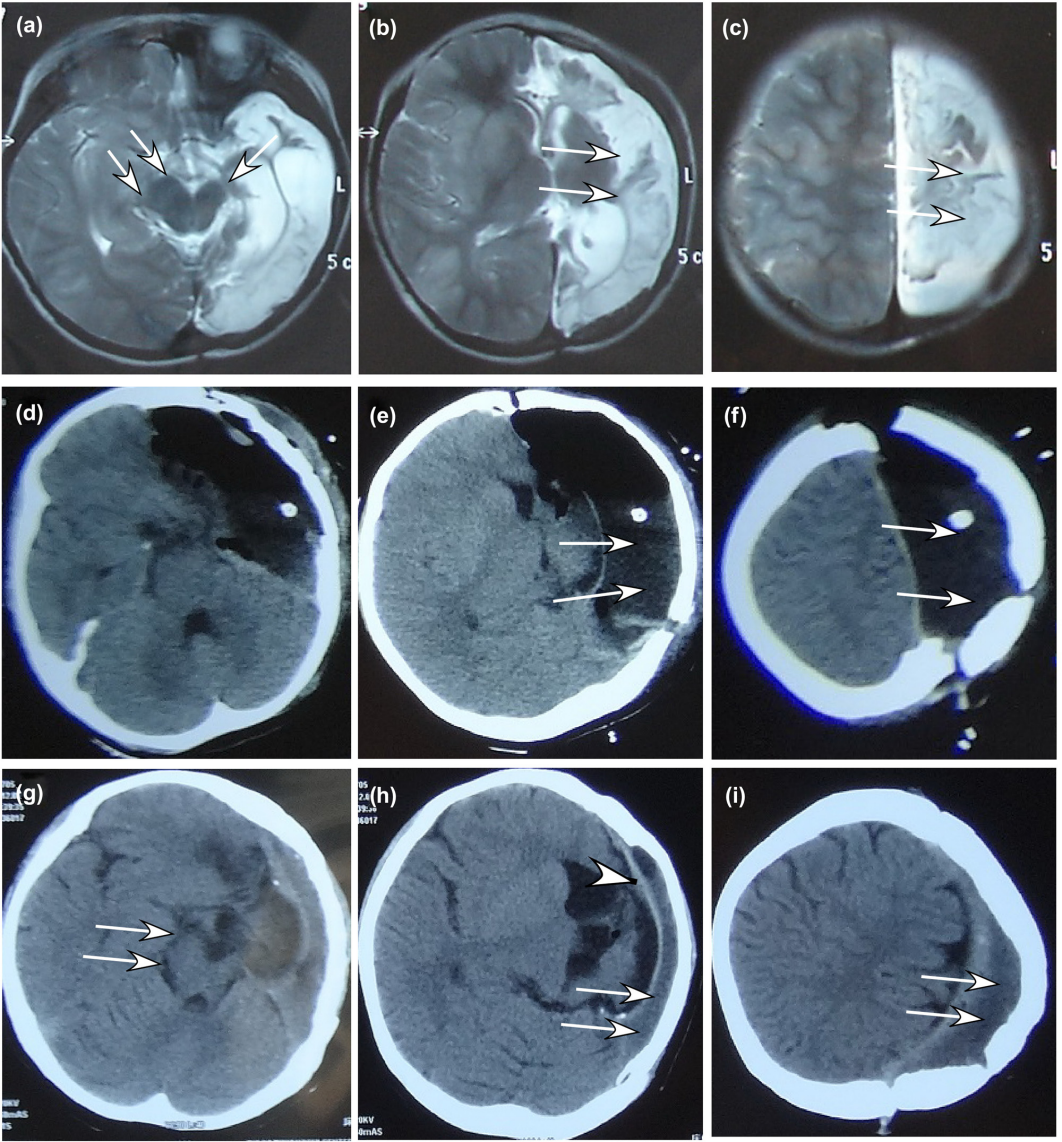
An 8-year-old girl with infantile hemiparesis and medically intractable epilepsy had undergone anatomical hemispherectomy. (a–c) Before the hemispherectomy, magnetic resonance imaging of the brain revealed compensatory enlargement (double arrows in A) of the right cerebral peduncle, atrophic left cerebral peduncle (single arrow in A), and atrophic brain tissue (double arrows in B and C). (d–f) CTS at 10 days posthemispherectomy demonstrated left-shifted midline structures and the residual intracranial space (double arrows in B and C) with accumulated air and fluid. (g–i) CTS 3 years later revealed enlarged cerebral peduncle on the right (double arrows in G), further left-shifted midline structures and shrank epidural space (double arrows in H and I) under the thickened skull. The arrow head indicates the dura mater separating the residual epidural space and the brain tissue on the right.
